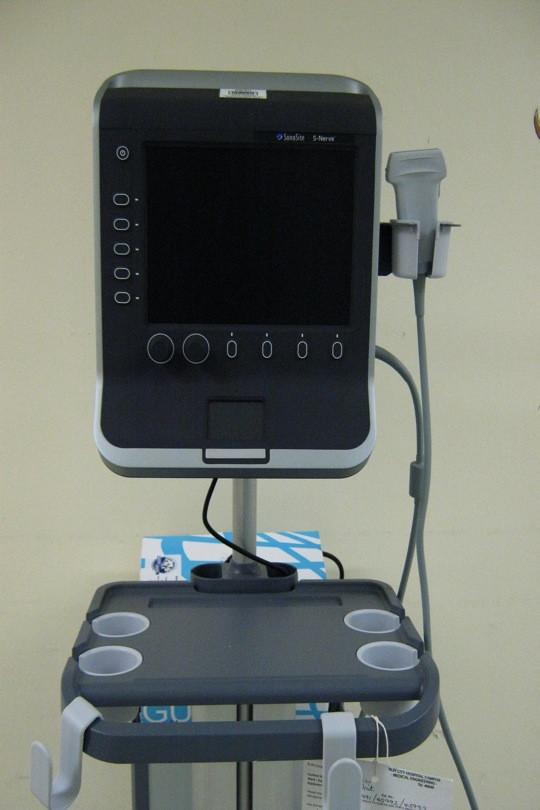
Sonosite S-Nerve Portable Ultrasound.
